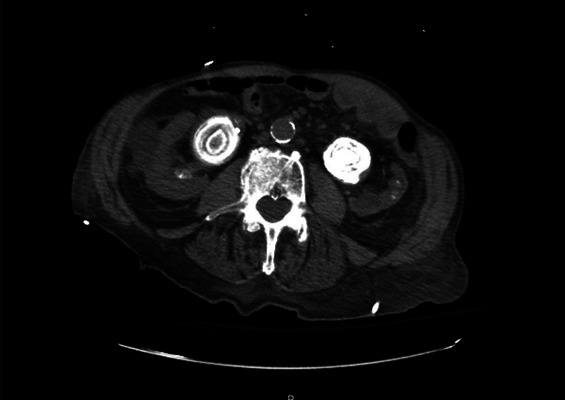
Noncontrast computed tomography coronal images demonstrate moderate left hydronephrosis from a large lamellated calculus within the renal pelvis measuring 5.0 cm. Moderate-to-severe right hydronephrosis secondary to large lamellated calculus within the right renal pelvis measuring 5.7 cm and an additional calculus within the proximal aspect of the right ureter measuring 1.8 cm.
